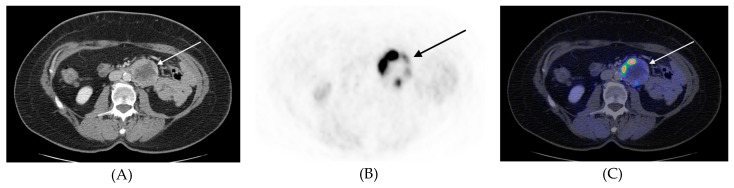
Transverse ^11^C-HED-PET/CT images, (A) CT, (B) PET, (C) PET/CT fusion, of patient #21 (Table 4) with a retroperitoneal paraganglioma left of the descending aorta (arrows). In this paraganglioma there was extended necrosis and merely peripheral tracer accumulation in the tumor.
